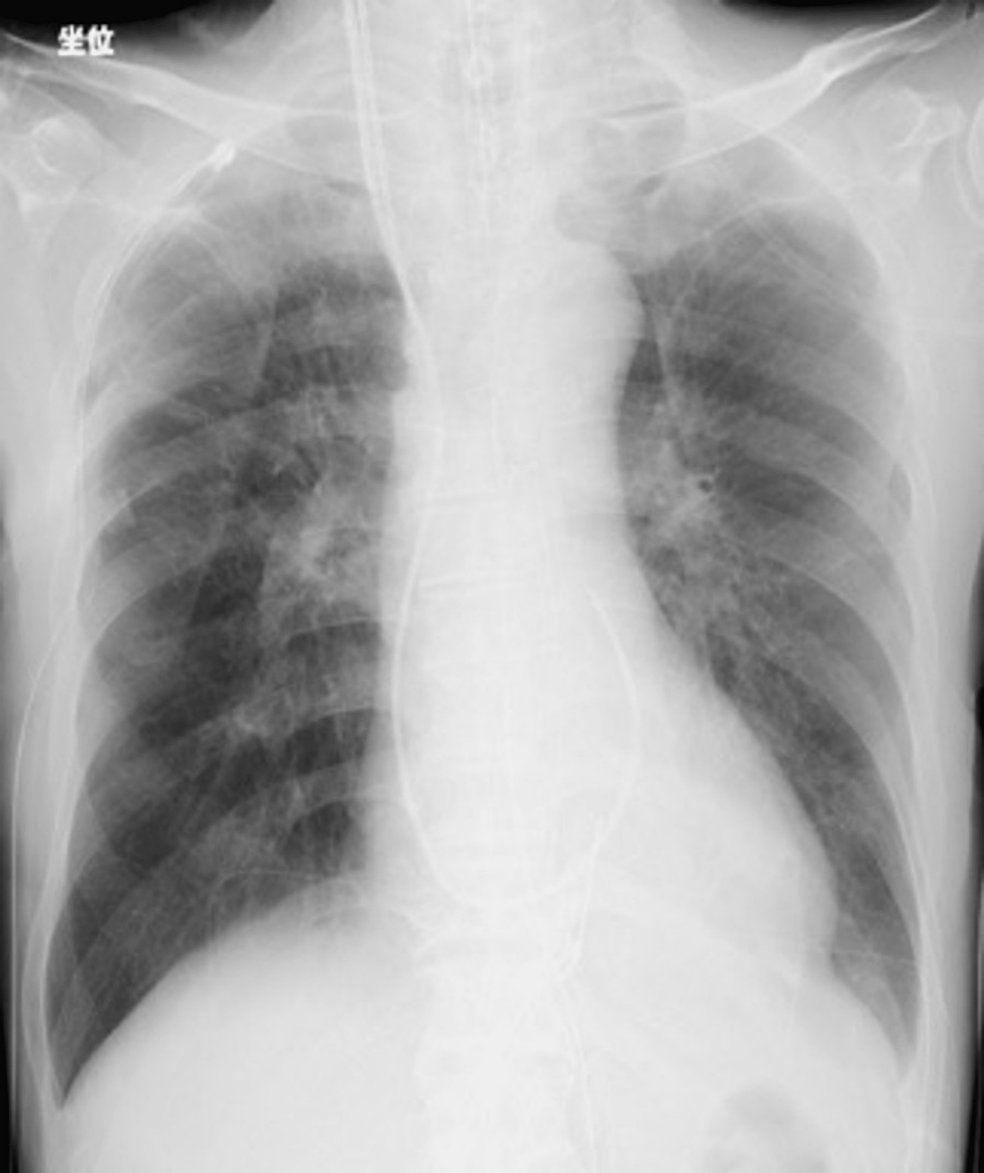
Chest radiograph on the second postoperative day. Improvement in both right pneumothorax and bilateral pulmonary edema was confirmed.
